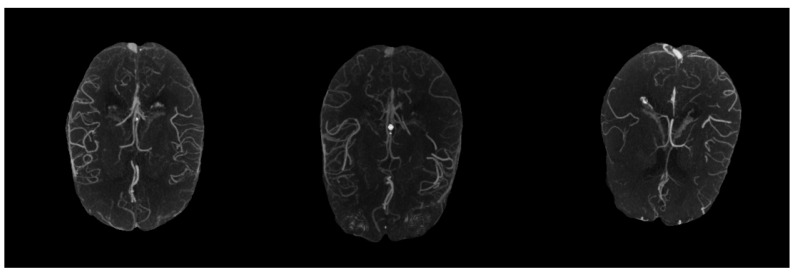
Examples of maximum intensity projection (MIP) images from 3D CTA image with different collateral scores. The left one is good collateral. The middle one is intermediate collateral. The right one is poor collateral.
